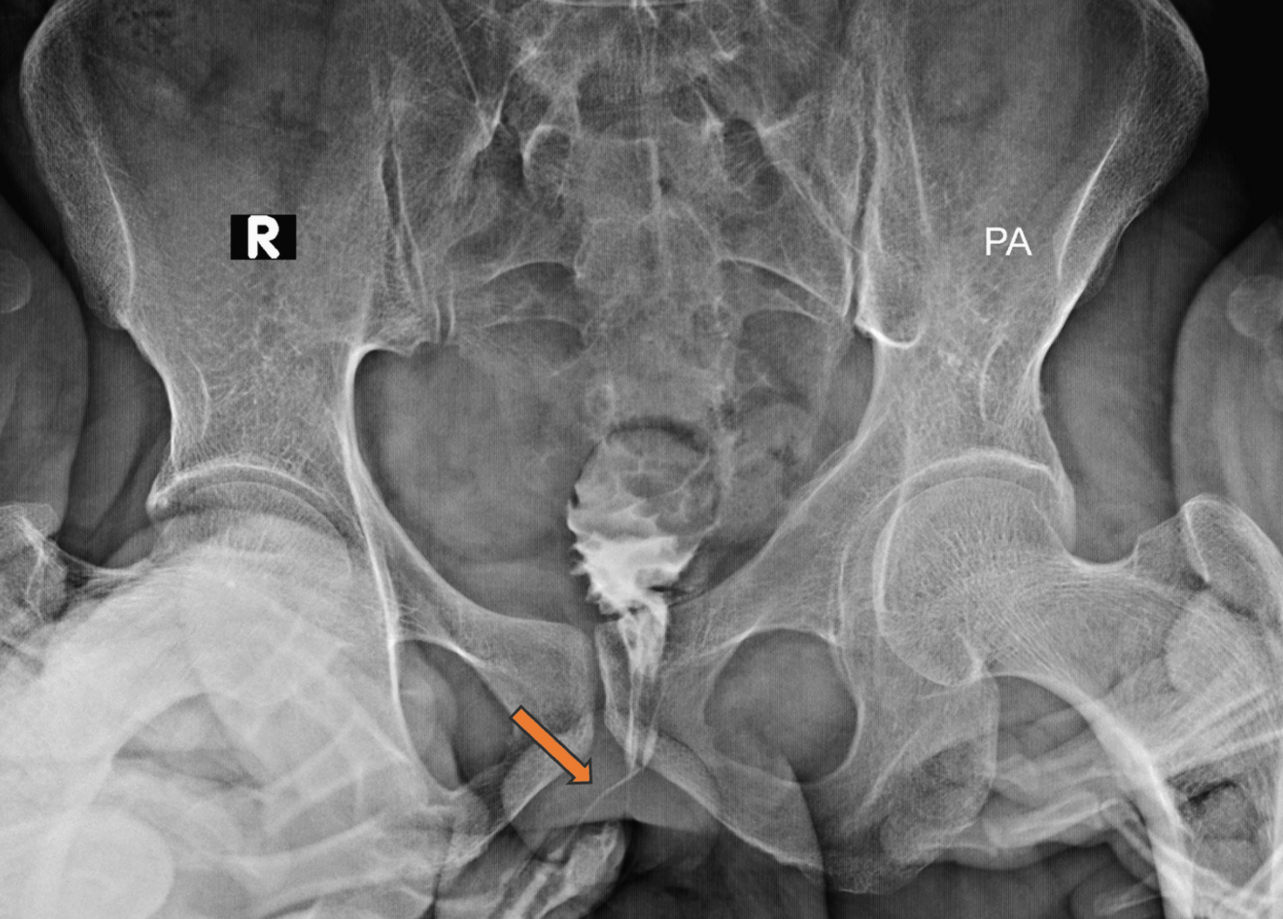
X-ray fistulogram showing contrast-filled perianal fistulous tract in PA view (orange arrow). The tract is outlined from the external opening toward the anal canal. Limited soft tissue detail highlights the modality’s constraints compared to MRI PA: posteroanterior; MRI: magnetic resonance imaging
